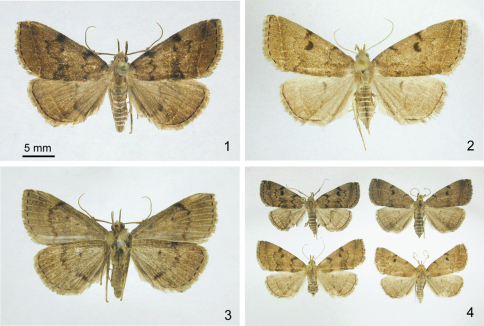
Zanclognatha dentata* sp. n. 1 Holotype male, dorsal. CT: Tolland Co., Mansfield 2 Female, dorsal. CT: Windham Co., Hampton Reservoir 3 Holotype male, ventral 4 Variation. NJ: Atlantic Co., Egg Harbor Township (upper left); CT: Windham Co., Sterling (upper right); CT: Windham Co., Hampton Reservoir (lower left); MA: Franklin Co., Montague, Plains Road (lower right).
